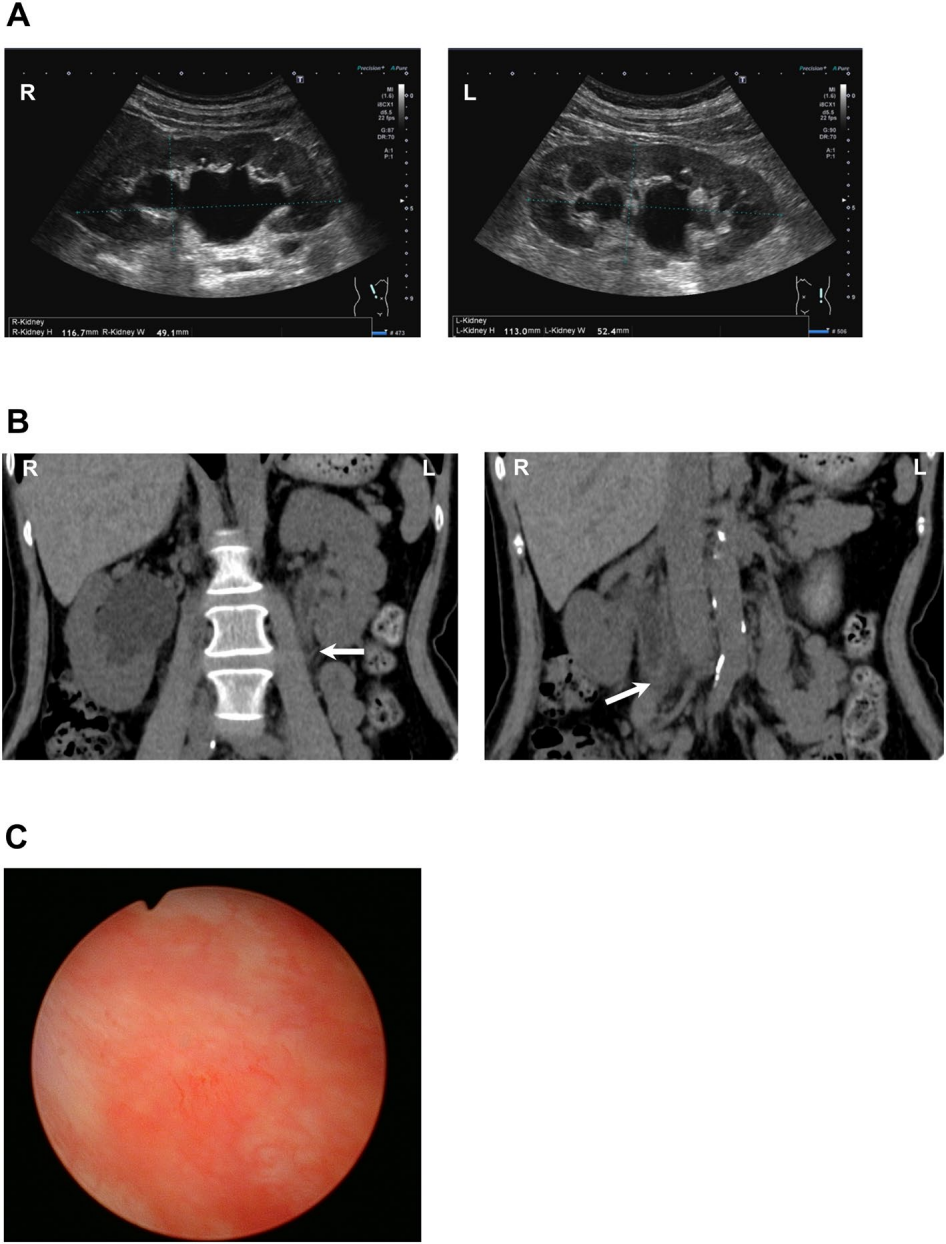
(A) Ultrasound imaging showing hydronephrosis of the (left image) right kidney and (right image) left kidney. (B) Computed tomography showing bilateral ureteral stenosis, as well as proximal ureteral strictures (arrows) with associated hydronephrosis in the bilateral upper urinary tract. (C) A Hunner lesion in the bladder.
